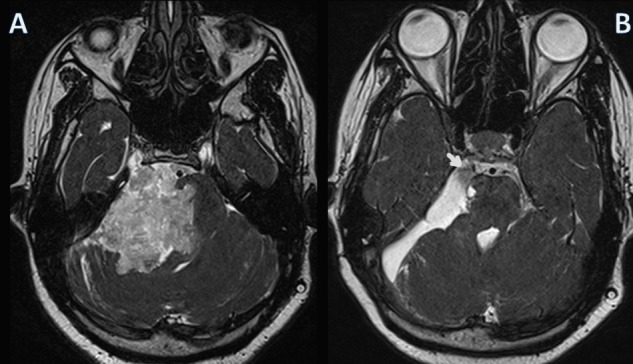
Preoperative and postoperative magnetic resonance imaging (MRI) Heavily T2-weighted MRI with thin slices (constructive interference in steady state) shows the preoperative lesion that had dug itself into the pontine surface (A). The postoperative MRI was taken three months after the surgery and it shows complete excision of the tumor (B). A flow related hypo-intensity is seen in the cerebrospinal fluid collection close to the fifth nerve (arrow). Removing the part of the tumor that was insinuating into the pontine surface caused mild and transient numbness in the trigeminal nerve distribution in the immediate postoperative period.
